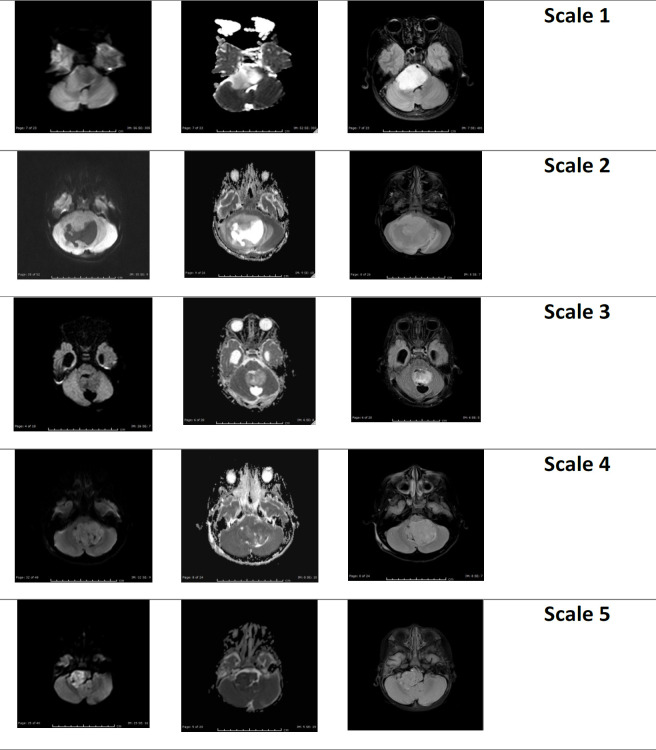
Different DWI Visual Scale. A, DWI visual scale 1; B, DWI visual scale 2; C, DWI visual scale 3; D, DWI visual scale 4; E, DWI visual scale 5
